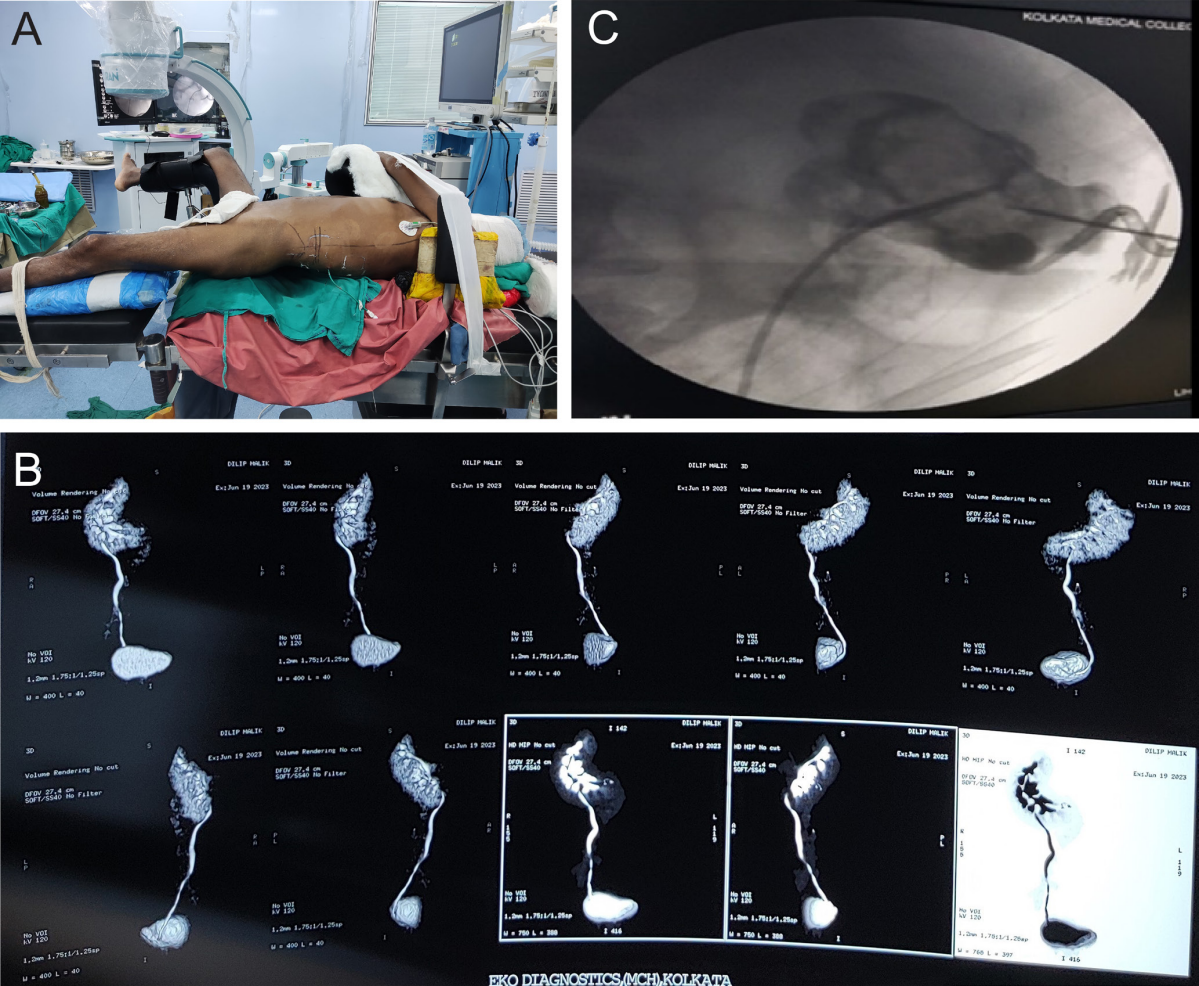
(A) Calcutta position in horseshoe kidney patient. (B) Preoperative computed tomography images of patients with horseshoe kidney. (C) Intraoperative fluoroscopic images of the patient.
